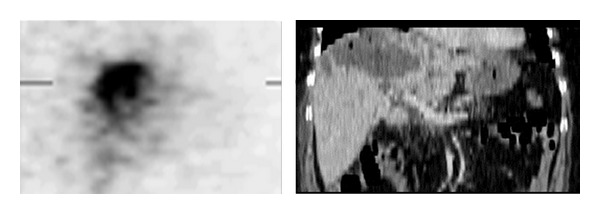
A patient with metastatic colon cancer treated with 296 MBq ^32^P particles. It shows a perfect concordance of Bremsstrahlung and CT images (grade 1).
